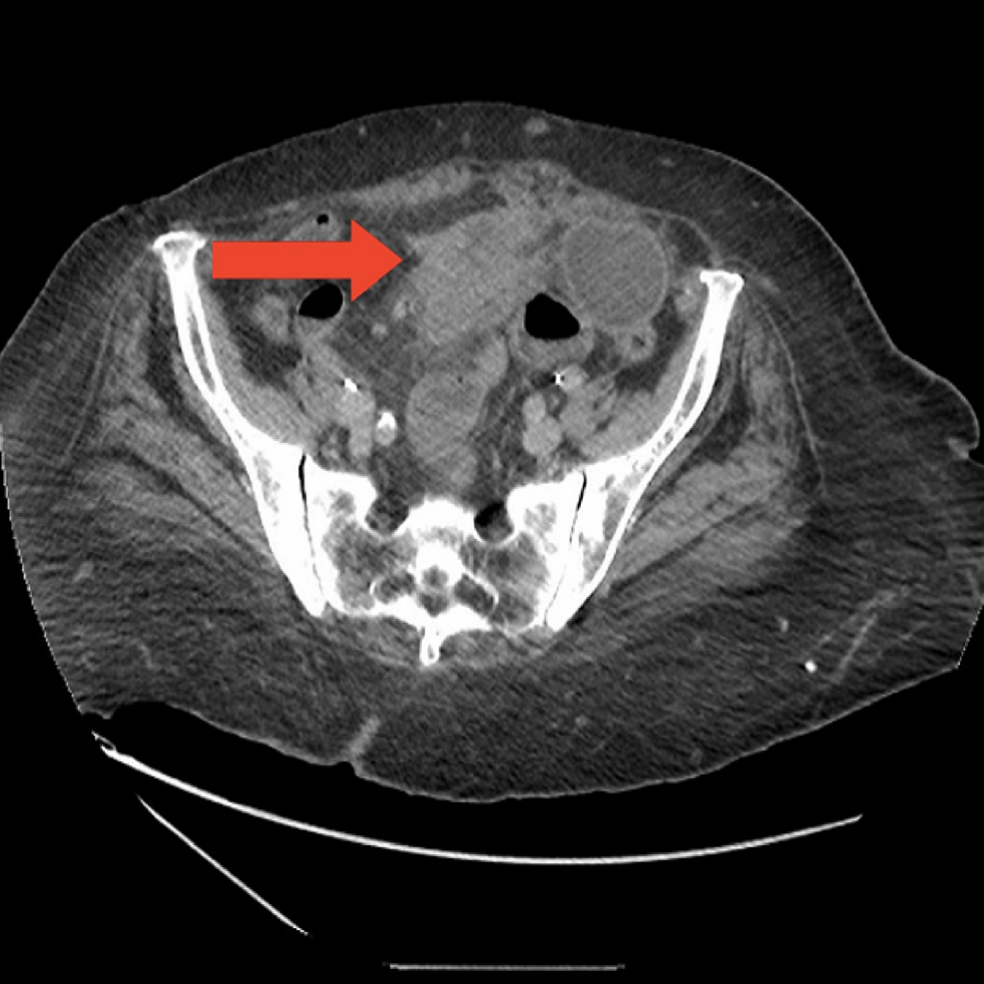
CT Axial view showing the mass (red arrow)
